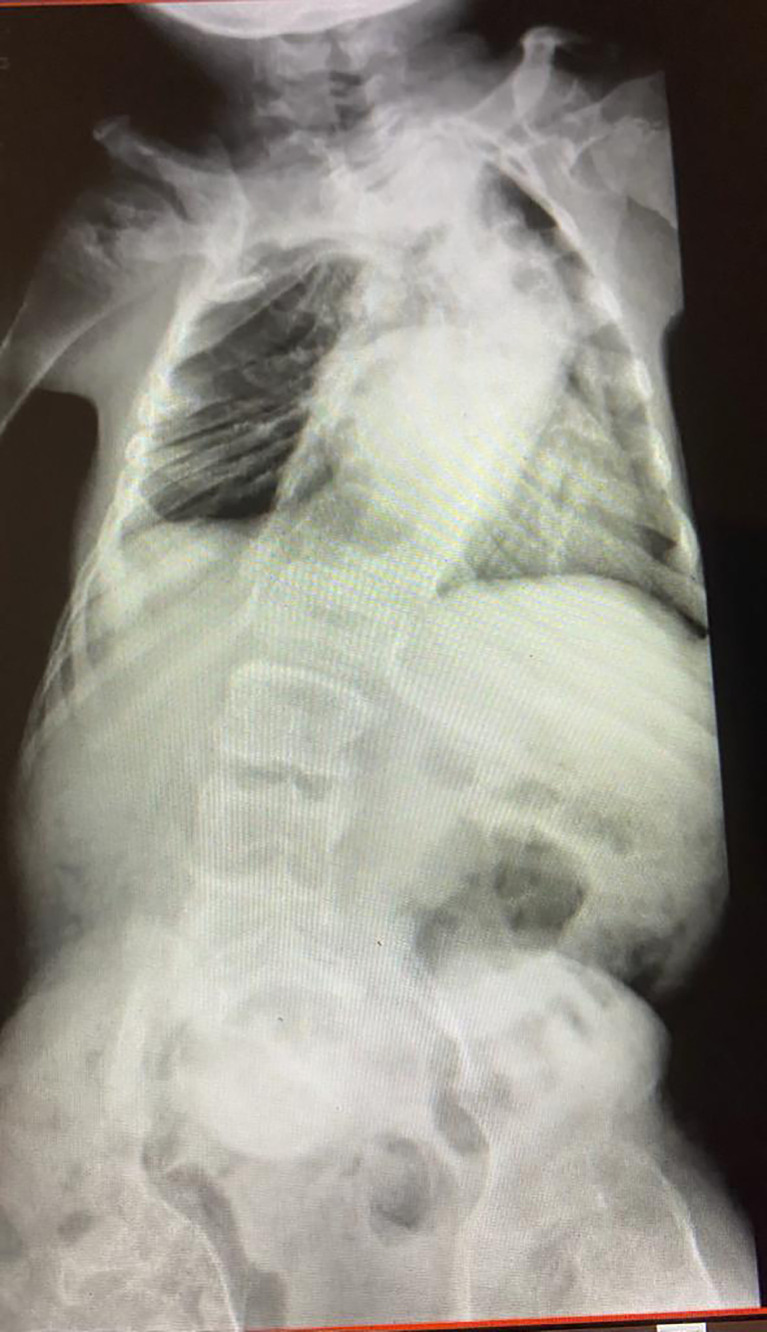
Whole spine X-ray of a patient with Osteogenesis Imperfecta and infected with COVID-19 showed severe scoliosis and Cobb’s angle of 70 in the thoracic curve.
